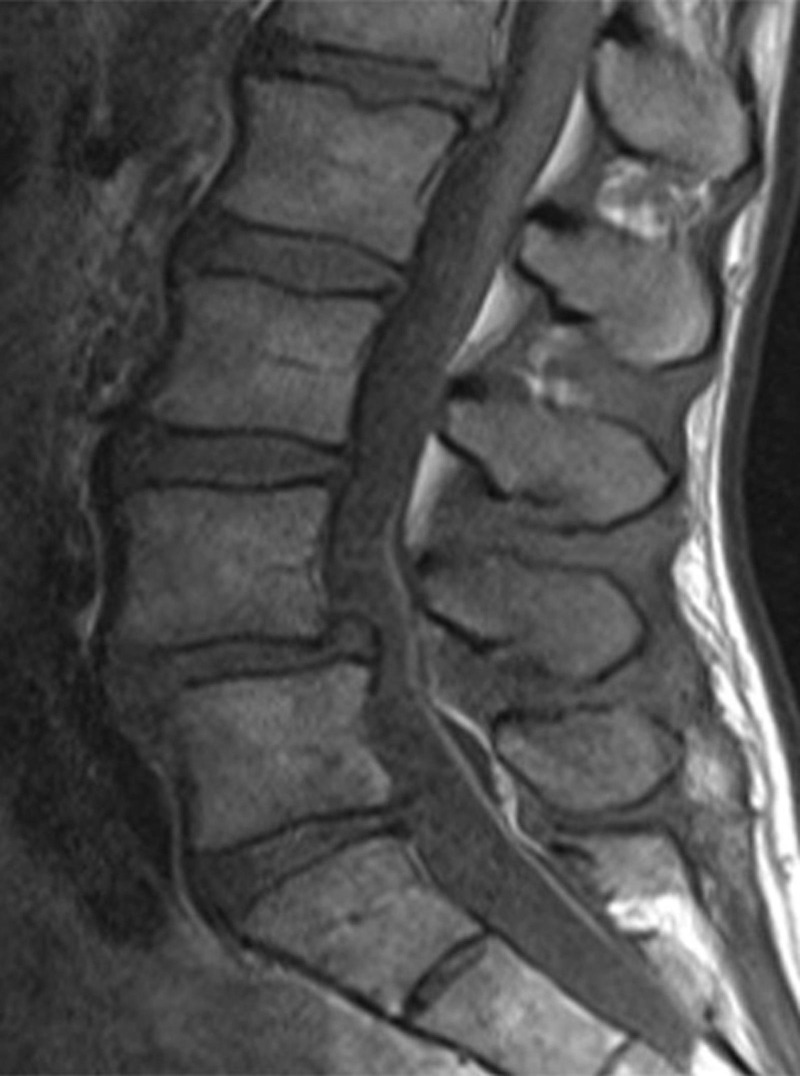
MRI of a Strong Candidate This MRI shows features of a good candidate for indirect decompression. Note the lack of central canal stenosis.
